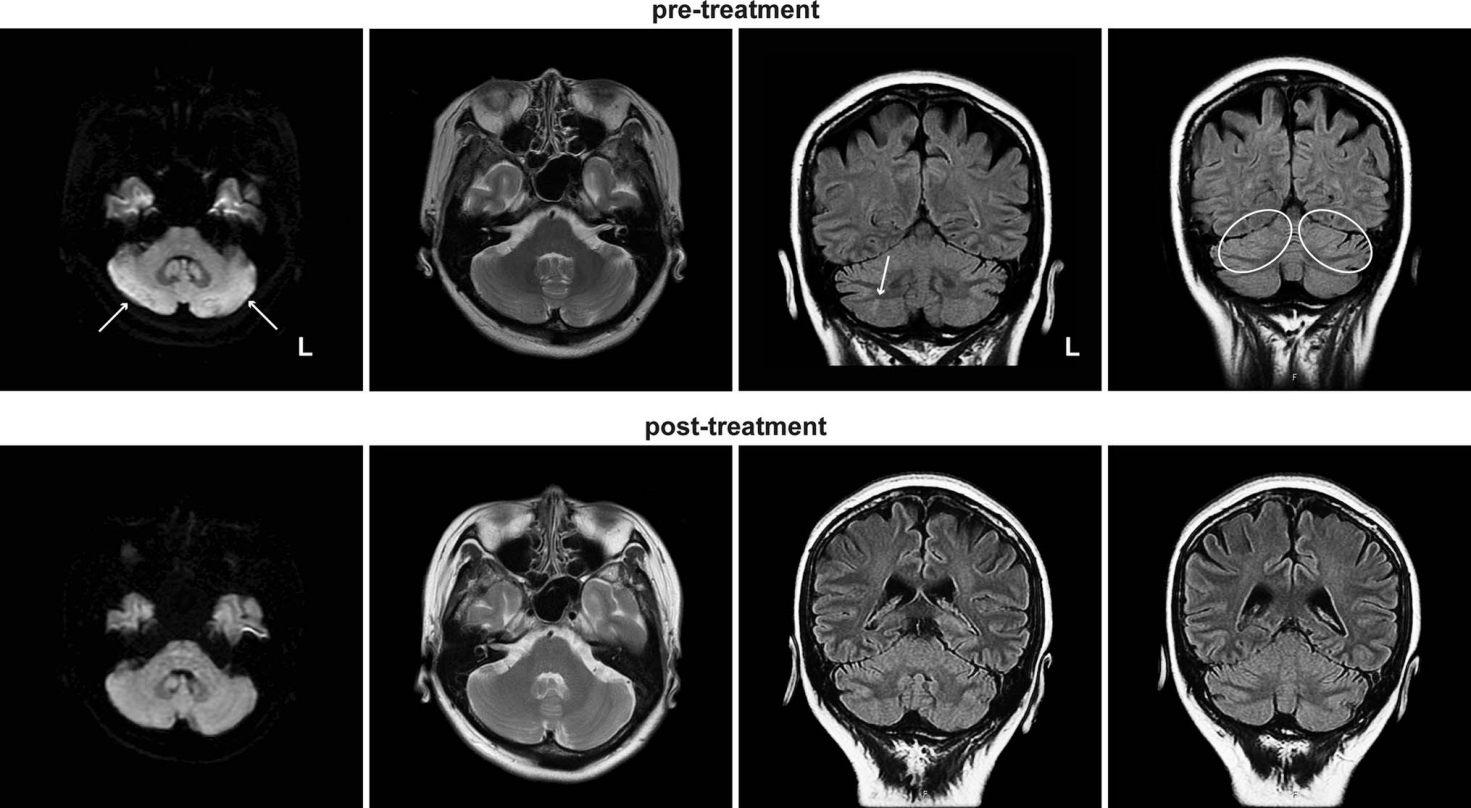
MRI findings of the patient. Axial DWI (left), axial T2-weighted image (middle left), and coronal FLAIR images (middle right and right) are shown. The hyperintensity in the cerebellum (arrows in DWI and FLAIR image) and edematous changes (circles in FLAIR image) was resolved after methylprednisolone treatments
